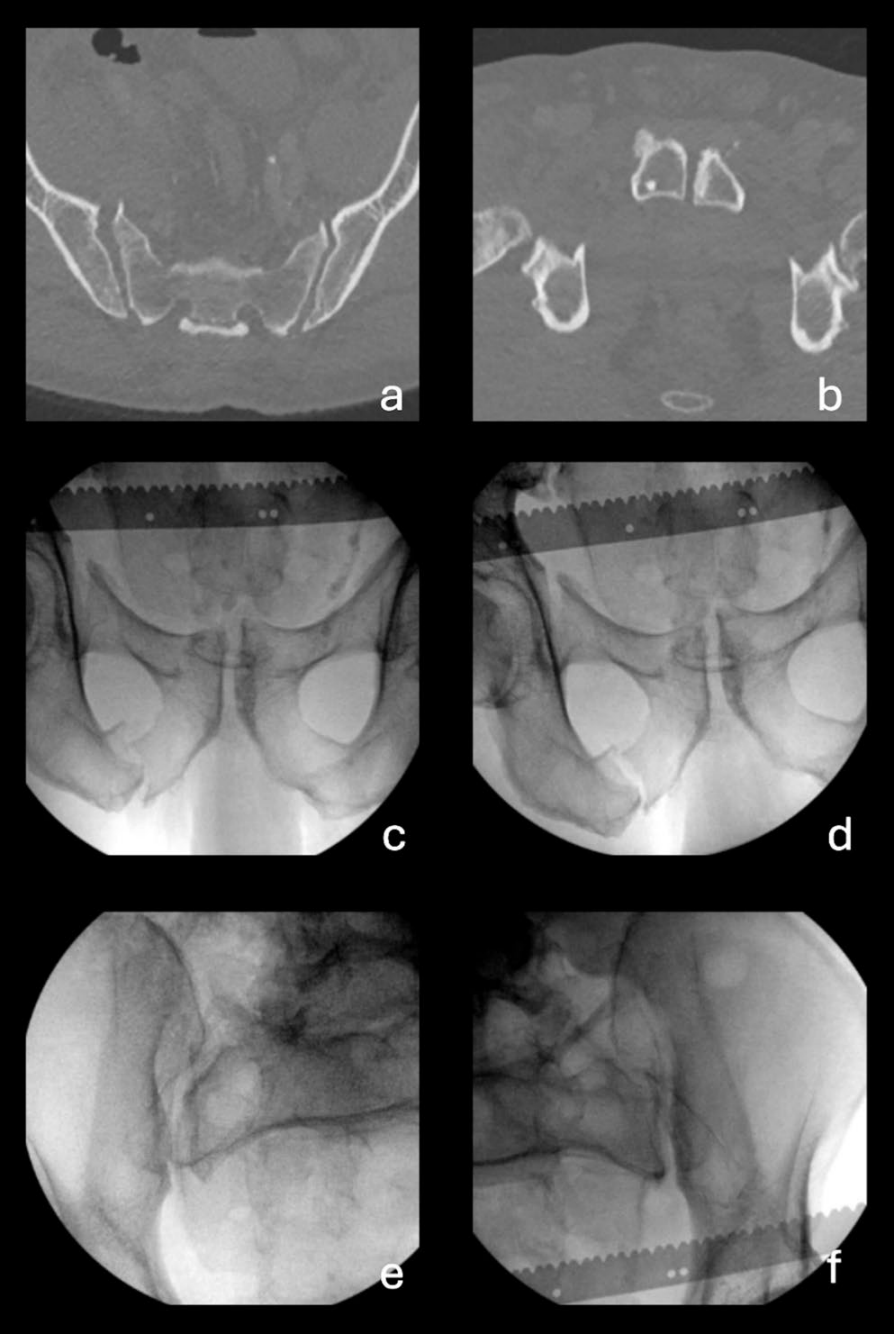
Case 32 is an example of a case when there is substantial sacroiliac (SI) joint diastasis, which is not reflected by the measurements of displacement in the anterior pelvis, potentially associated with fractures of the superior and inferior pubic rami (a) axial CT scan of the sacrum without pelvic binder on, showing diastasis in the right SI joint (b) axial CT image of pubic symphysis showing minimal symphyseal diastasis (c) anterior-posterior (AP) examination under anaesthesia (EUA) image of the pubic symphysis in the external rotation stress maneuver, showing 0.8 cm of symphyseal diastasis (d) AP EUA image of the pubic symphysis in the push/pull stress maneuver, showing 0.5 cm of rotational instability (e) Obturator inlet EUA image of right SI joint in the external rotation stress maneuver, demonstrating complete SI diastasis (f) Obturator inlet EUA image of left SI joint in the external rotation stress maneuver, showing no SI diastasis
